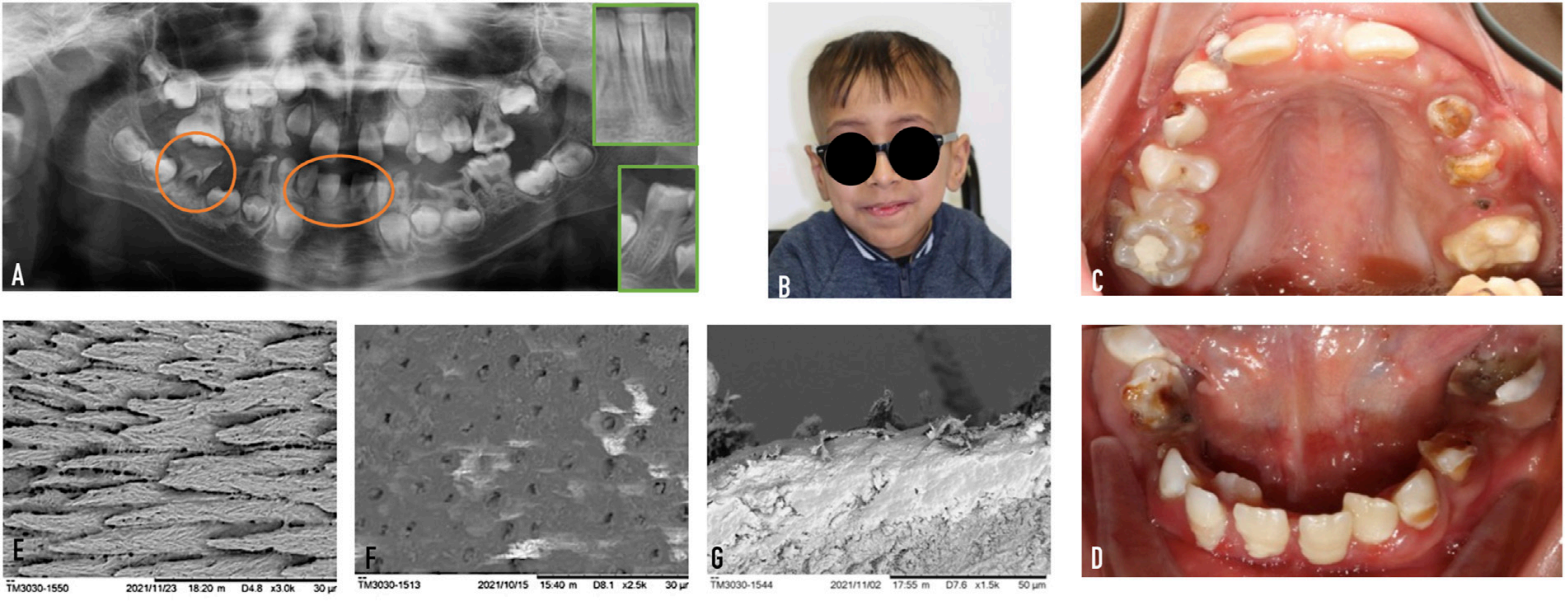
Case report. (A) Orthopantomogram X-ray of our 10-year-old Singleton-Merten patient. In orange, a first permanent molar (tooth n°46) and central permanent mandibular incisors (teeth n° 31–41) with short roots were highlighted. To compare, a healthy patient’s teeth are shown in a green insert. (B) Photography of the patient. (C,D) Intra-oral photographies of the maxillary and the mandibular arch. (E) First molar enamel. (F) Second temporary molar dentin. (G) First molar cement.
